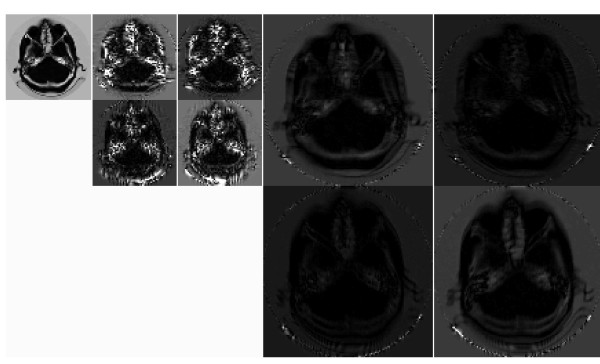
The contourlet transform of a CT brain image.
